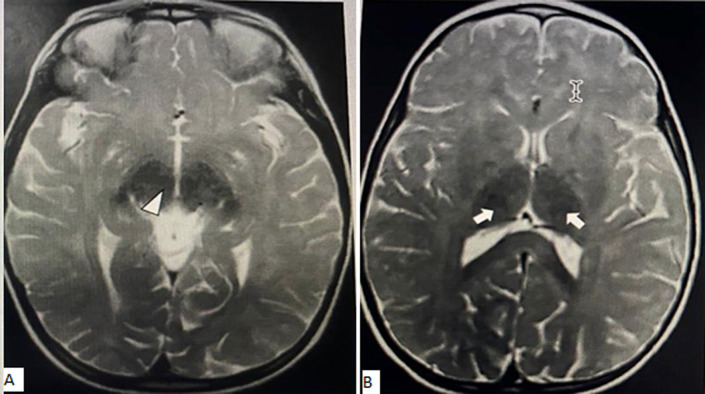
(A,B) brain magnetic resonance imaging thalamic (arrow) and diencephalic (arrow ahead) low signal T2
